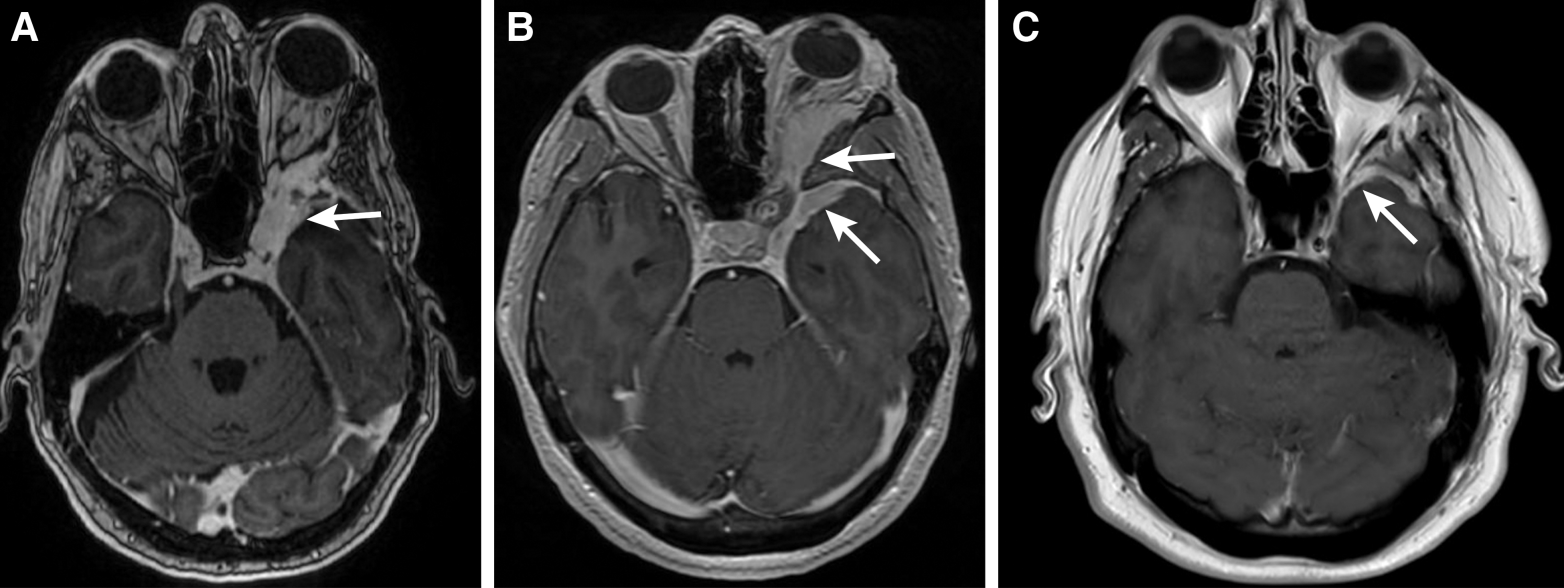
Representative axial T1-weighted contrast-enhanced magnetic resonance images of the brain from 3 patients with a spheno-orbital meningioma (SOM): (A) SOM invading the left orbital apex (*arrow*), with an extension into the orbit causing proptosis. (B) A left-sided SOM showing dural thickening and signal enhancement, with progressive growth toward the orbit (*arrows*). (C) A small left-sided SOM showing dural thickening and increased signal intensity, without evidence of intraorbital extension (*arrow*). Used with permission from Barrow Neurological Institute, Phoenix, Arizona.
